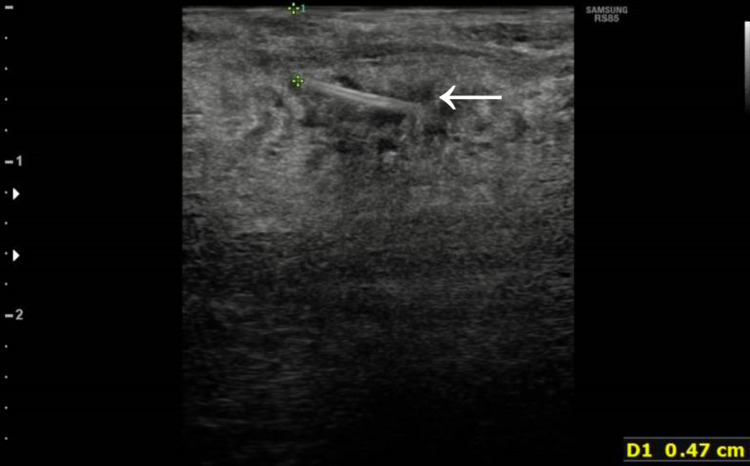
Ultrasound scan still image of intraglossal foreign body (<1mm diameter, 9mm long, 4.7mm deep at the most superficial aspect) White arrow points to the suspected fish bone
